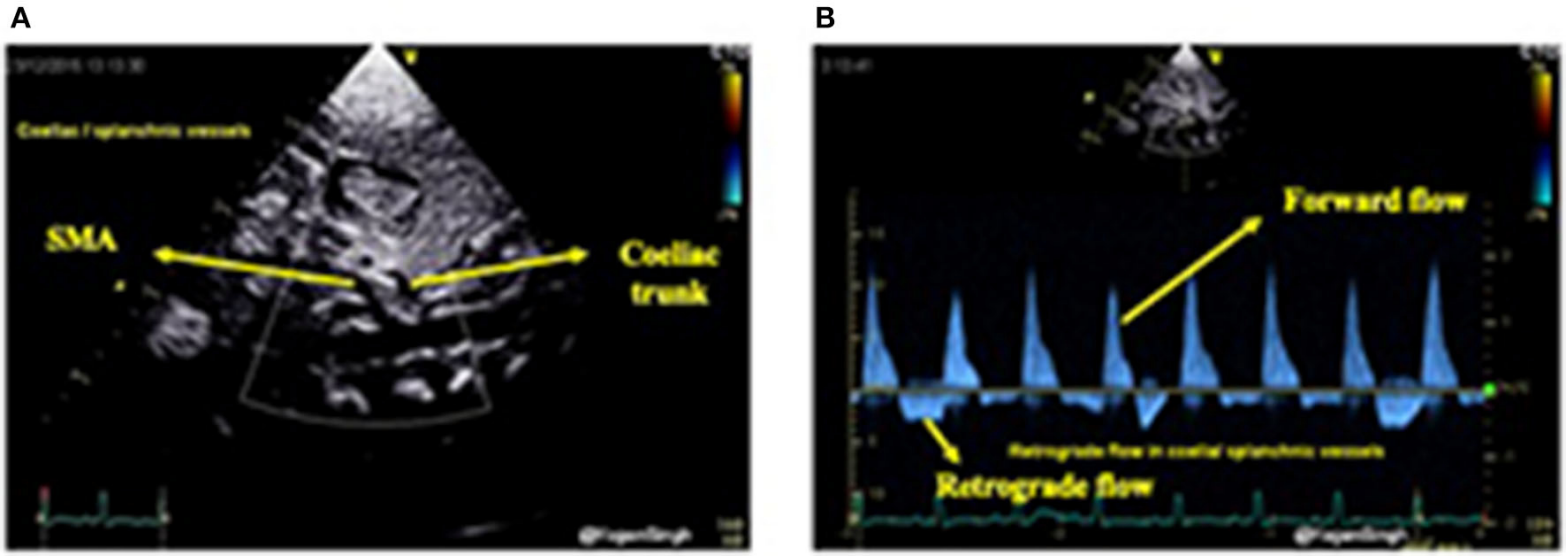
Color flow mapping and Doppler assessment of blood flow in the coeliac trunk and superior mesenteric artery in sub-costal sagittal view. (A) Showing color flow mapping of the coeliac and superior mesenteric arteries; and (B) showing retrograde blood flow during diastole in the the coeliac and superior mesenteric arteries indicating “ductal steal” in presence of a large PDA.
